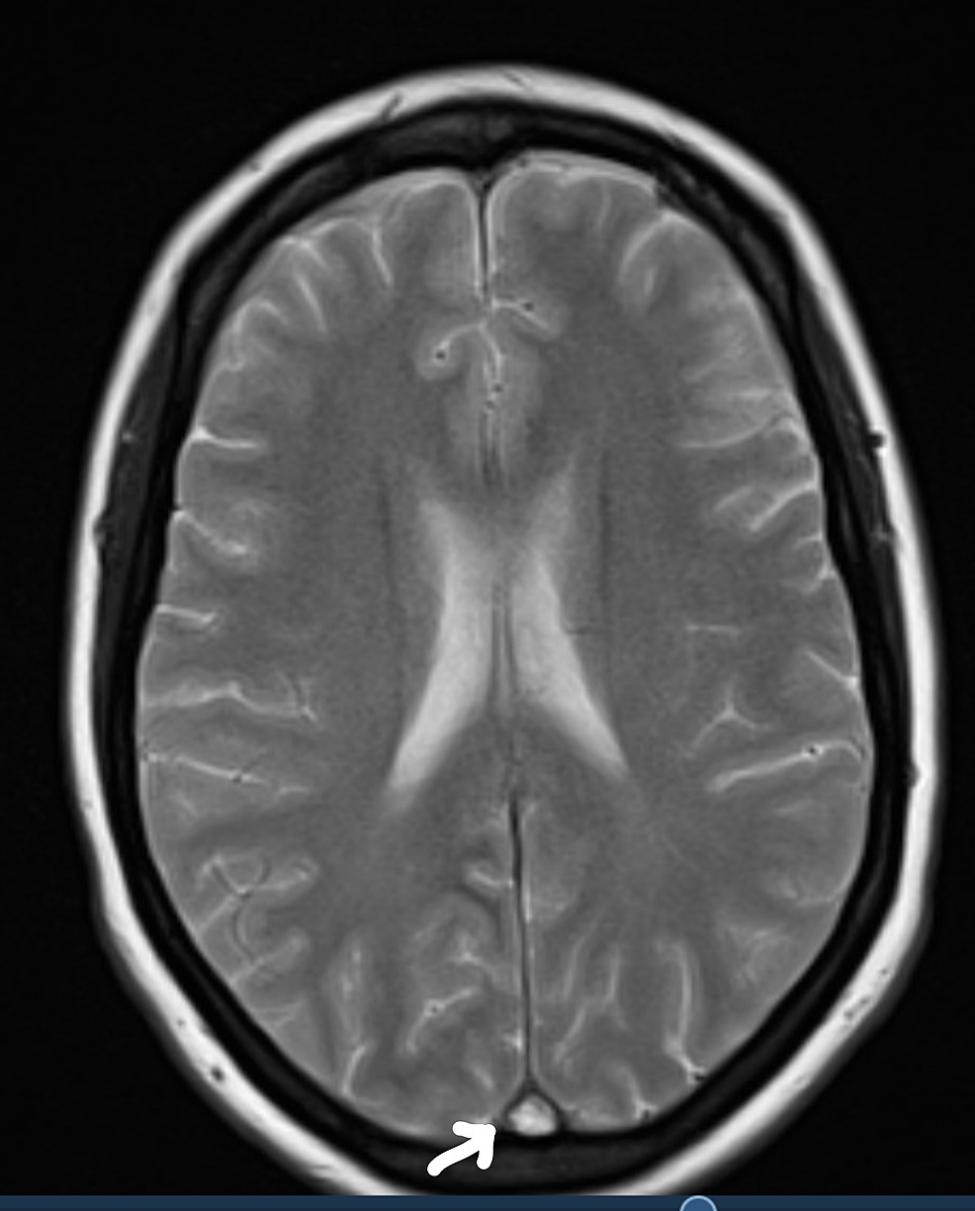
MRI head showing cerebral venous thrombosis
